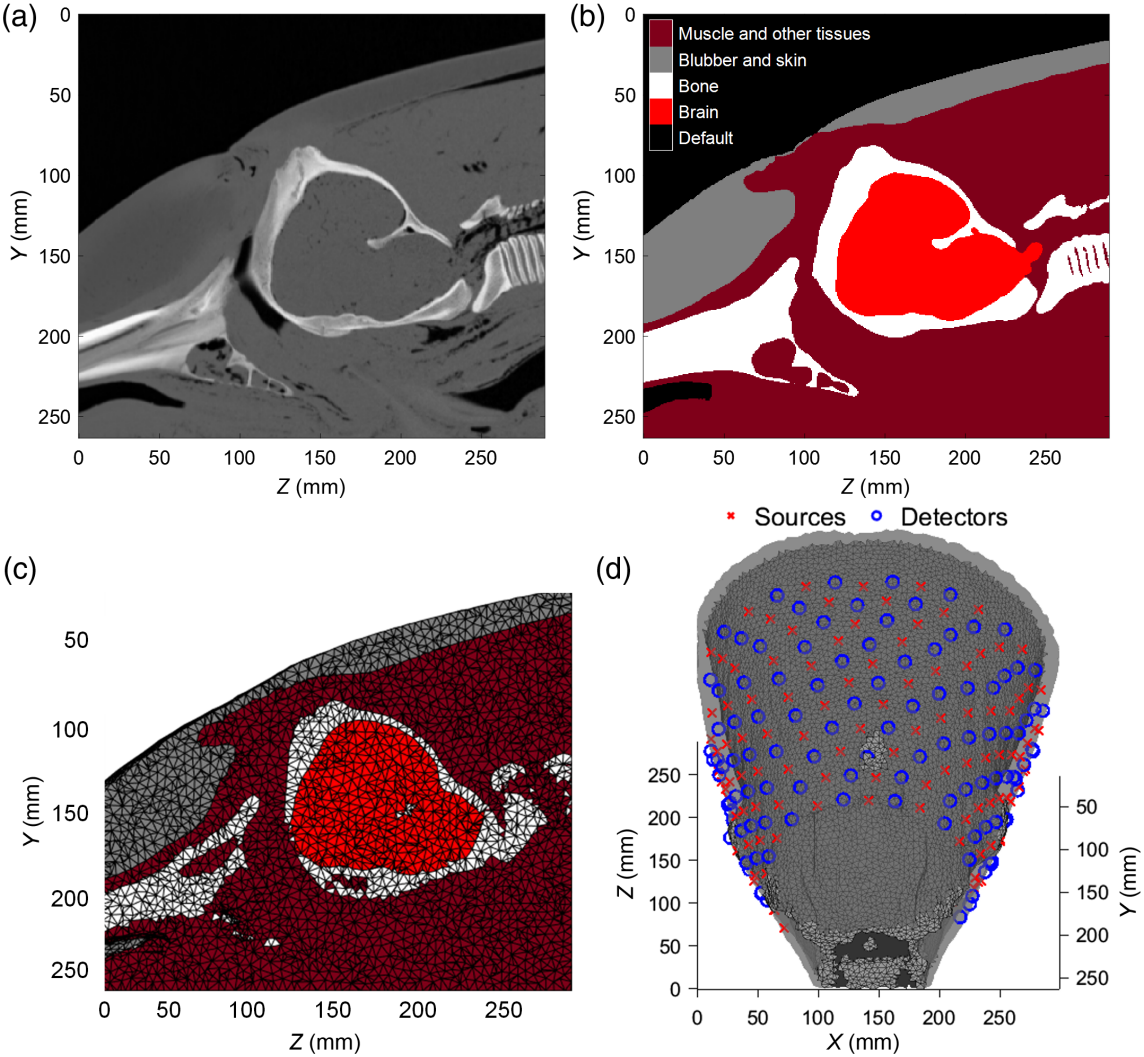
Mesh generation. (a) Sagittal plane cross-section of the CT scan used to generate the model. (b) Manually assigned tissue labels for the cross-section shown in (a). (c) Mesh generated from the tissue labels: blubber and skin (gray), muscle, and other tissues layer (dark red), bone (white), and the brain (light red). Black lines are the edges of tetrahedrons inside the mesh. (d) The same mesh as in (c) in non-opaque grayscale. Blue circles show the location of detectors, and red crosses show source locations.
